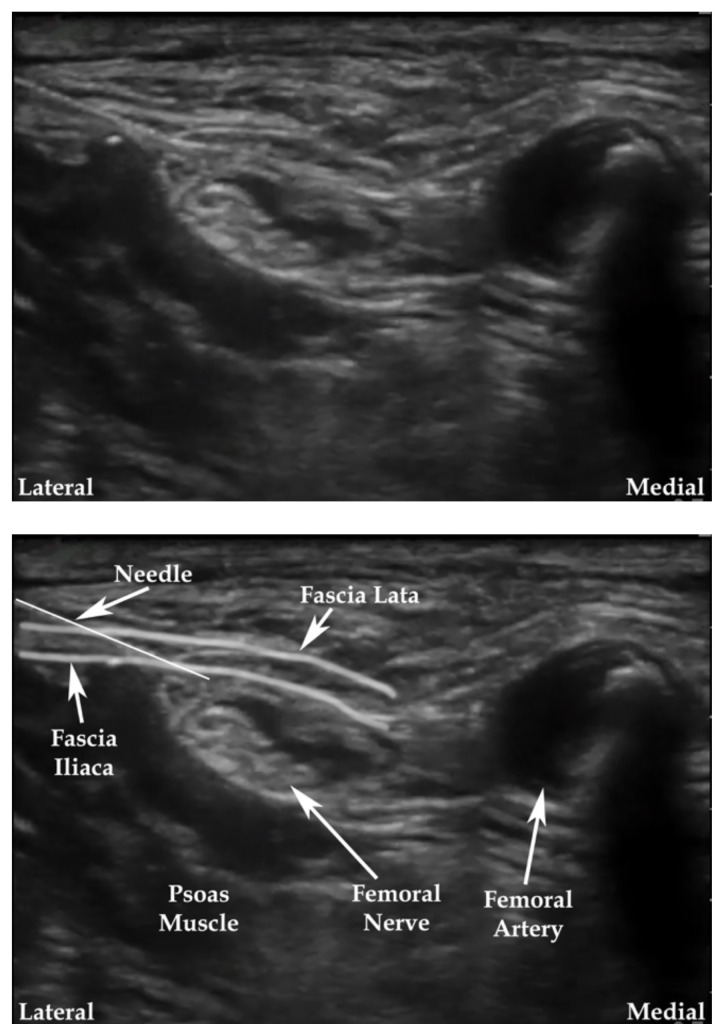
Ultrasound-guided femoral nerve block. Modified from source.^15^
